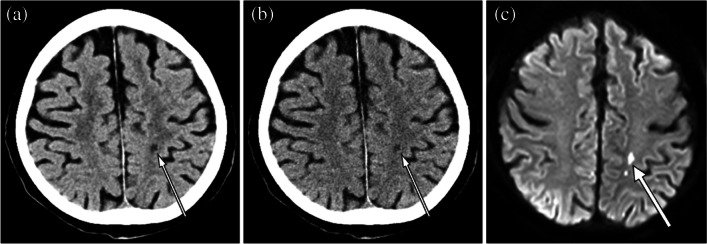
Unenhanced head CT (a, b) of a 65-year-old female patient with acute infarct in the left sup-MCA region (white arrows). The time interval from onset to CT examination was 9 h. The conspicuity of this acute infarct was rated as 5, 4, and 5 in DLR (a) and 4, 3, and 3 in Hybrid IR (b) by readers 1, 2, and 3, respectively. Window level / width is 25 / 50 Hounsifield unit for both (a) and (b). Diffusion-weighted image is also shown in (c)
